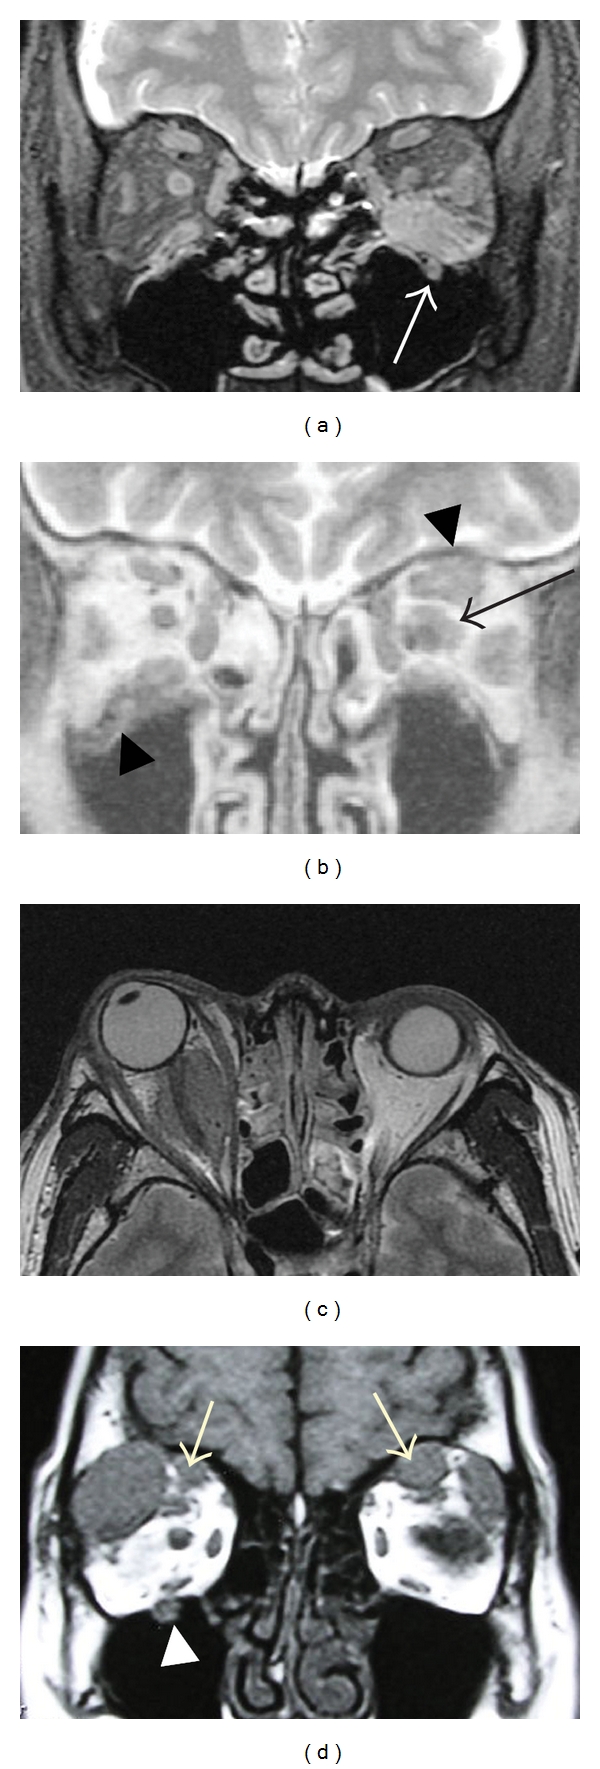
Representative cases with IgG4-related orbital inflammatory lesions other than lacrimal glands. (a) Enlargements in the left inferior rectus muscle and infraorbital nerve (arrow) in a 65-year-old man with a serum IgG4 of 404 mg/dL (case number 7 in Table 1). (b) Swelling of the left superior and lateral rectus muscles, a mass lesion around the left optic disc (arrow), and enlargements of the left supraorbital nerve and the right infraorbital nerve (arrow heads) were seen in a 60-year-old man with a serum IgG4 of 463 mg/dL (case number 8). (c) Mass lesion around the right optic disc was observed in a 44-year-old man with a serum IgG4 of 599 mg/dL (case number 11). (d) Bilateral supraorbital nerve enlargements (arrows) and right infraorbital nerve enlargement were observed in a 47-year-old woman with a serum IgG4 of 1000 mg/dL (case number 16). Magnetic resonance images are T1-weighted in (b) and (d) and T2-weighted in (a) and (c).
